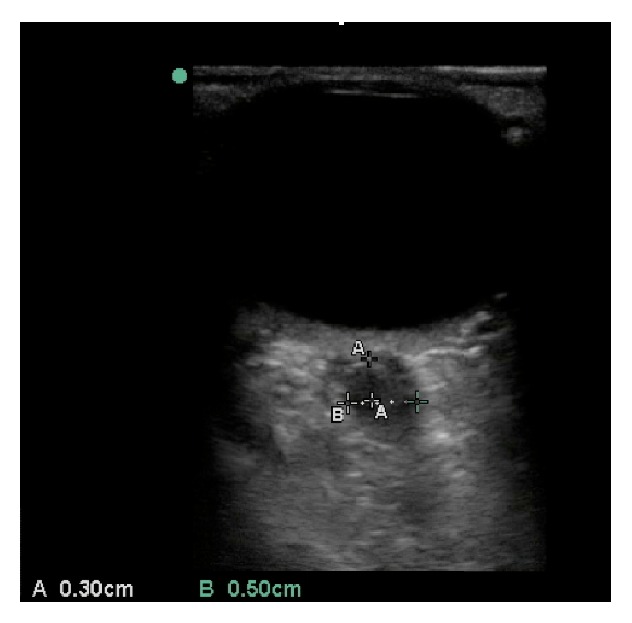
Bedside ocular ultrasound of the patient's left eye demonstrating optic nerve sheath diameter (ONSD) measurement. The measurement of the ONSD is taken 3 mm posterior to the globe and occurs perpendicular to the long axis of the optic nerve.
